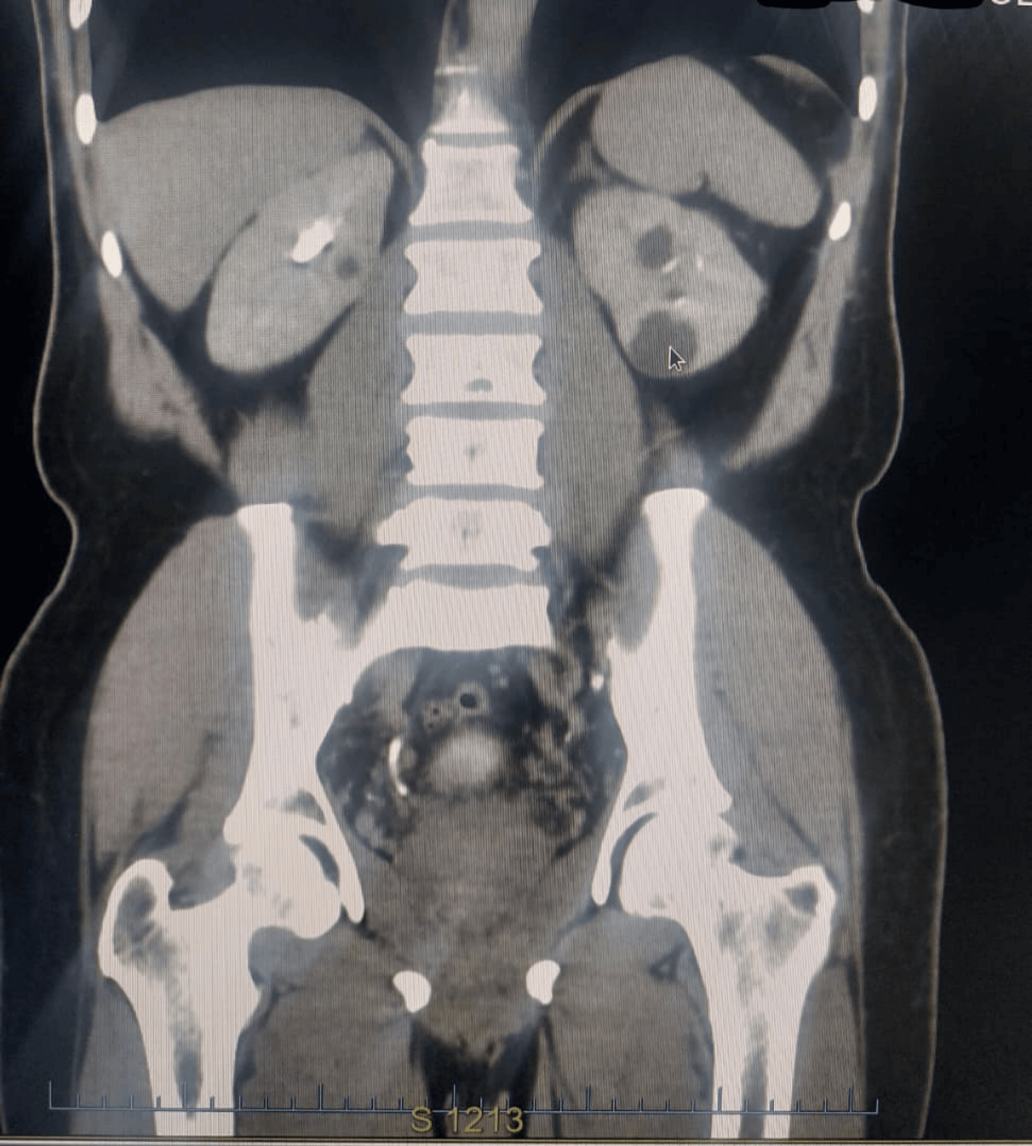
Abdominopelvic CT scan prior to nephrectomy
